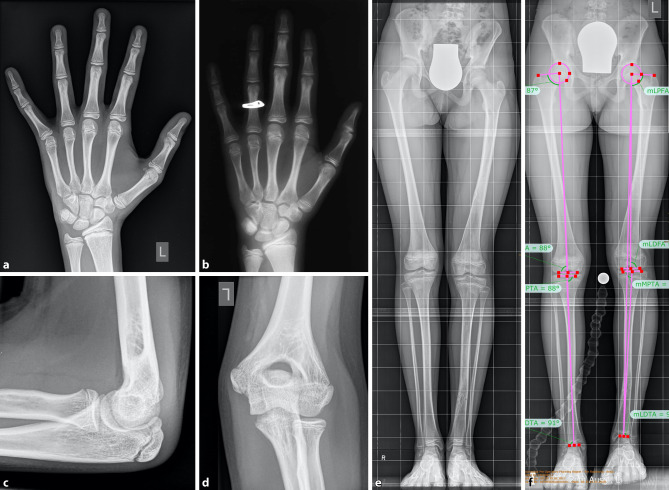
Example of bone age determination. A girl with chronological age of 12 years and 10 months. a Left hand X‑ray and b reference X‑ray according to the Greulich and Pyle atlas for a bone age of 11 years in girls. c Lateral and d antero/posterior (a/p) left elbow X‑ray to determine the bone age according to the Sauvegrain method. The evaluation results in 24.5 points corresponding to a bone age of 11.5 years in girls. The difference between the girls chronological age and the bone age is > 1 year speaking for a retarded bone age. e Full standing a/p bilateral lower extremity radiograph with a leg length discrepancy of −2 cm on the left side. f Preoperative X‑ray with 2 cm block under the left foot to equalize leg length discrepancy, and limb alignment measurements
